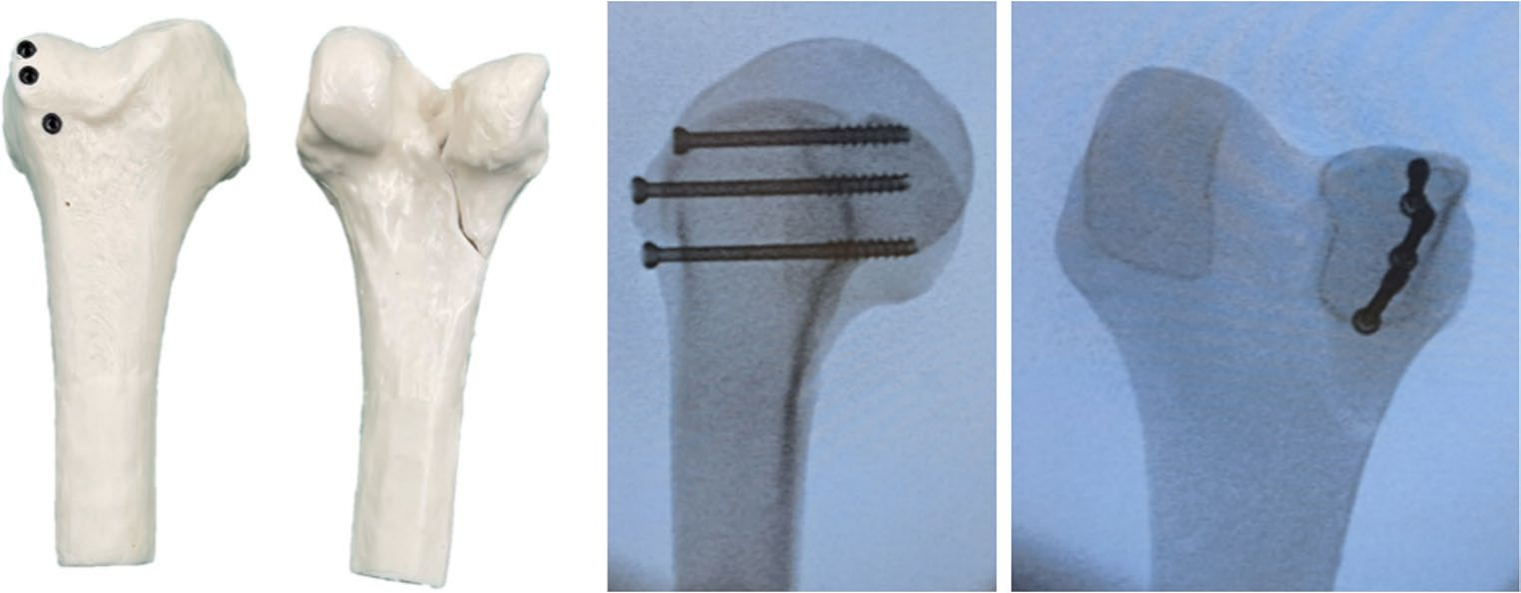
Distal femur with a lateral Hoffa fracture treated using three parallel 5 mm cannulated screws inserted in an anterior-to-posterior direction (left). Postoperative radiograph in medial to lateral (middle) and anterior to posterior (right) projection
